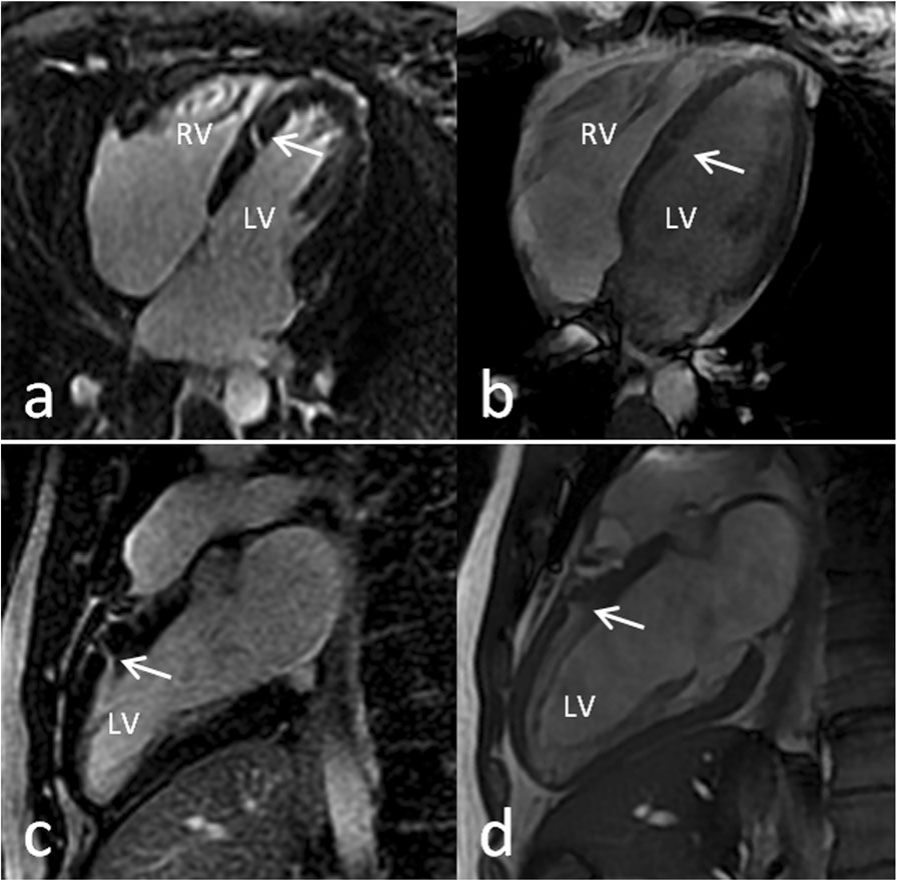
Representative cardiac magnetic resonance images revealing left ventricular clefts. Late gadolinium enhancement images (a and c) and cine sequences (b and d) of two patients with Wilson’s disease. The arrows show a deep left ventricular cleft in the interventricular septum (patient 1, upper row) and in the anterior wall (patient 2, lower row). LV - left ventricle, RV - right ventricle
